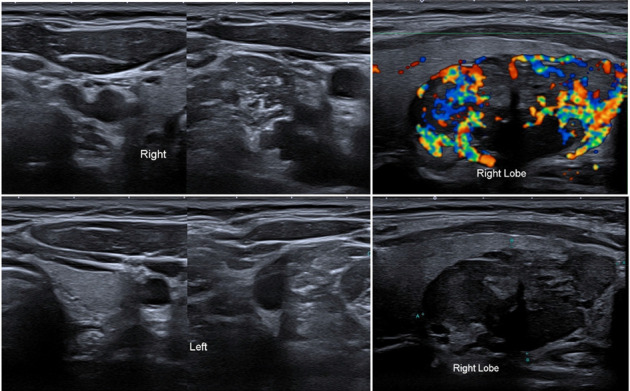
Ultrasound of the left and right lobe of the thyroid.
